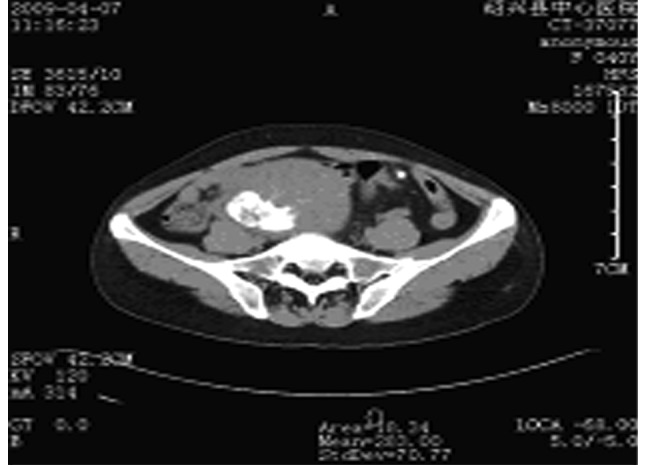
CT (computed tomography) showing a large irregular, lobulated mass in the lower abdomen and pelvis. The mass was ~13.2×13.5 cm with a large number of article sheets and an area of calcification density shadowing.
